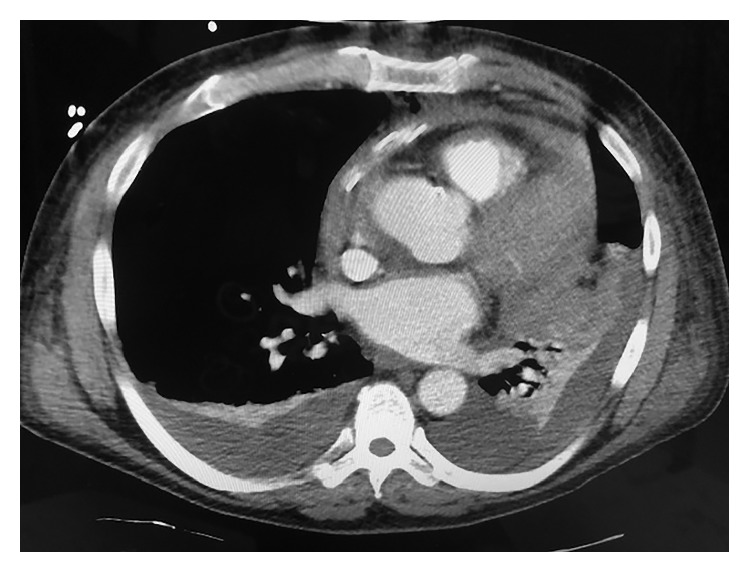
Computed tomography (CT) scan of the chest with intravenous contrast, which reveals a very large pericardial effusion, compressing the right and left ventricles and the right atrium.
